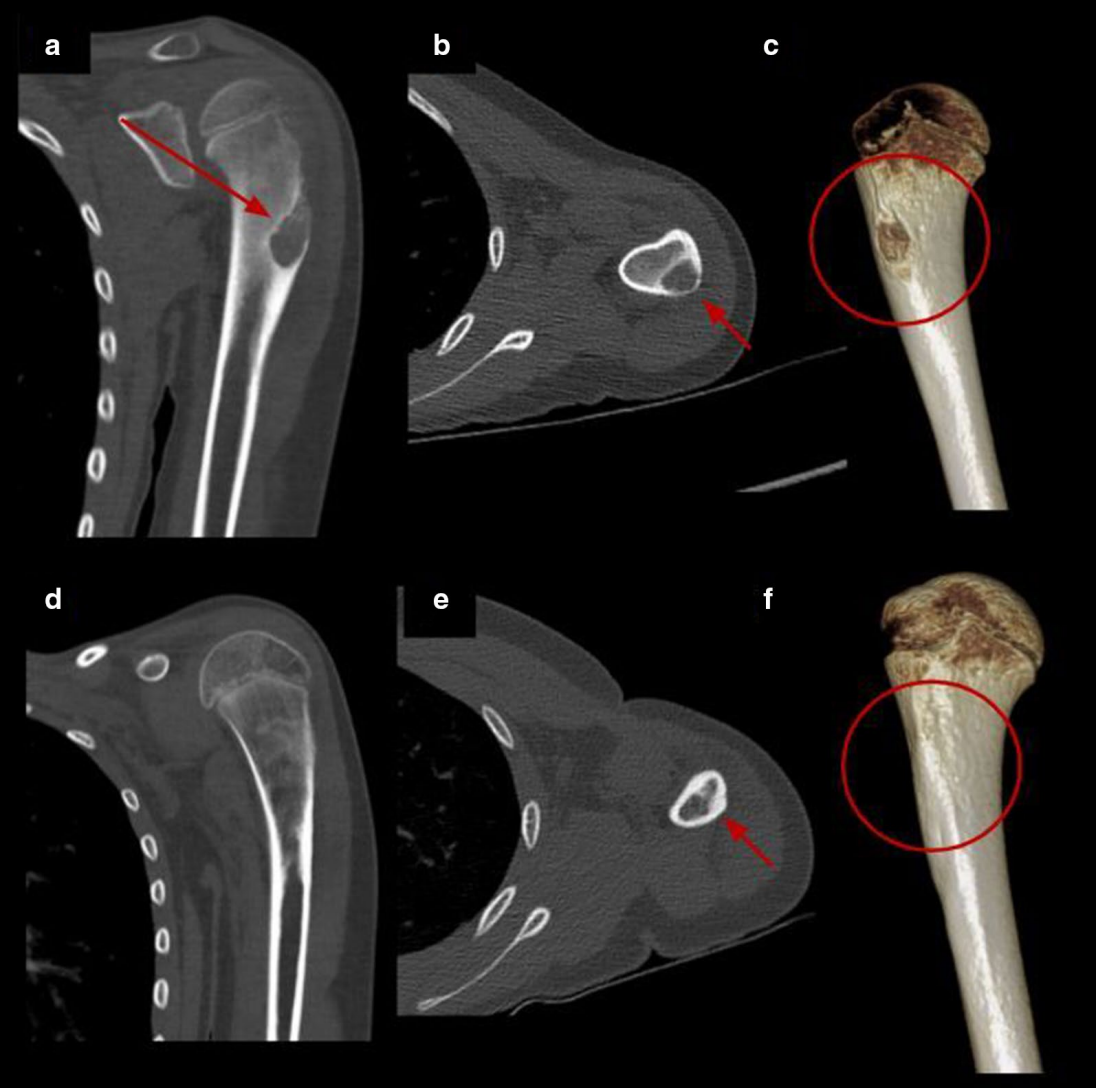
Percutaneous cryoablation of humeral osteoblastoma in a 9-y.o patient. a–c Coronal, axial CT images and volume rendering reconstruction of the left humerus show a lytic lesion consistent with osteoblastoma (red arrow and red circle). d–f Control CT 7 months after treatment with complete resolution of the lesion (red arrow and red circle)
